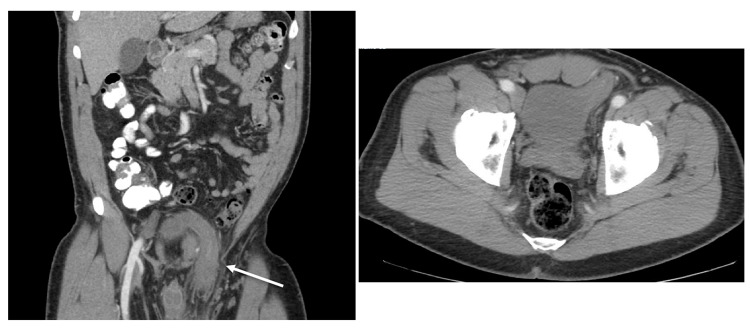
CT demonstrating inclusion of urinary bladder in inguinoscrotal hernia with coronal (left) and axial (right) views.
